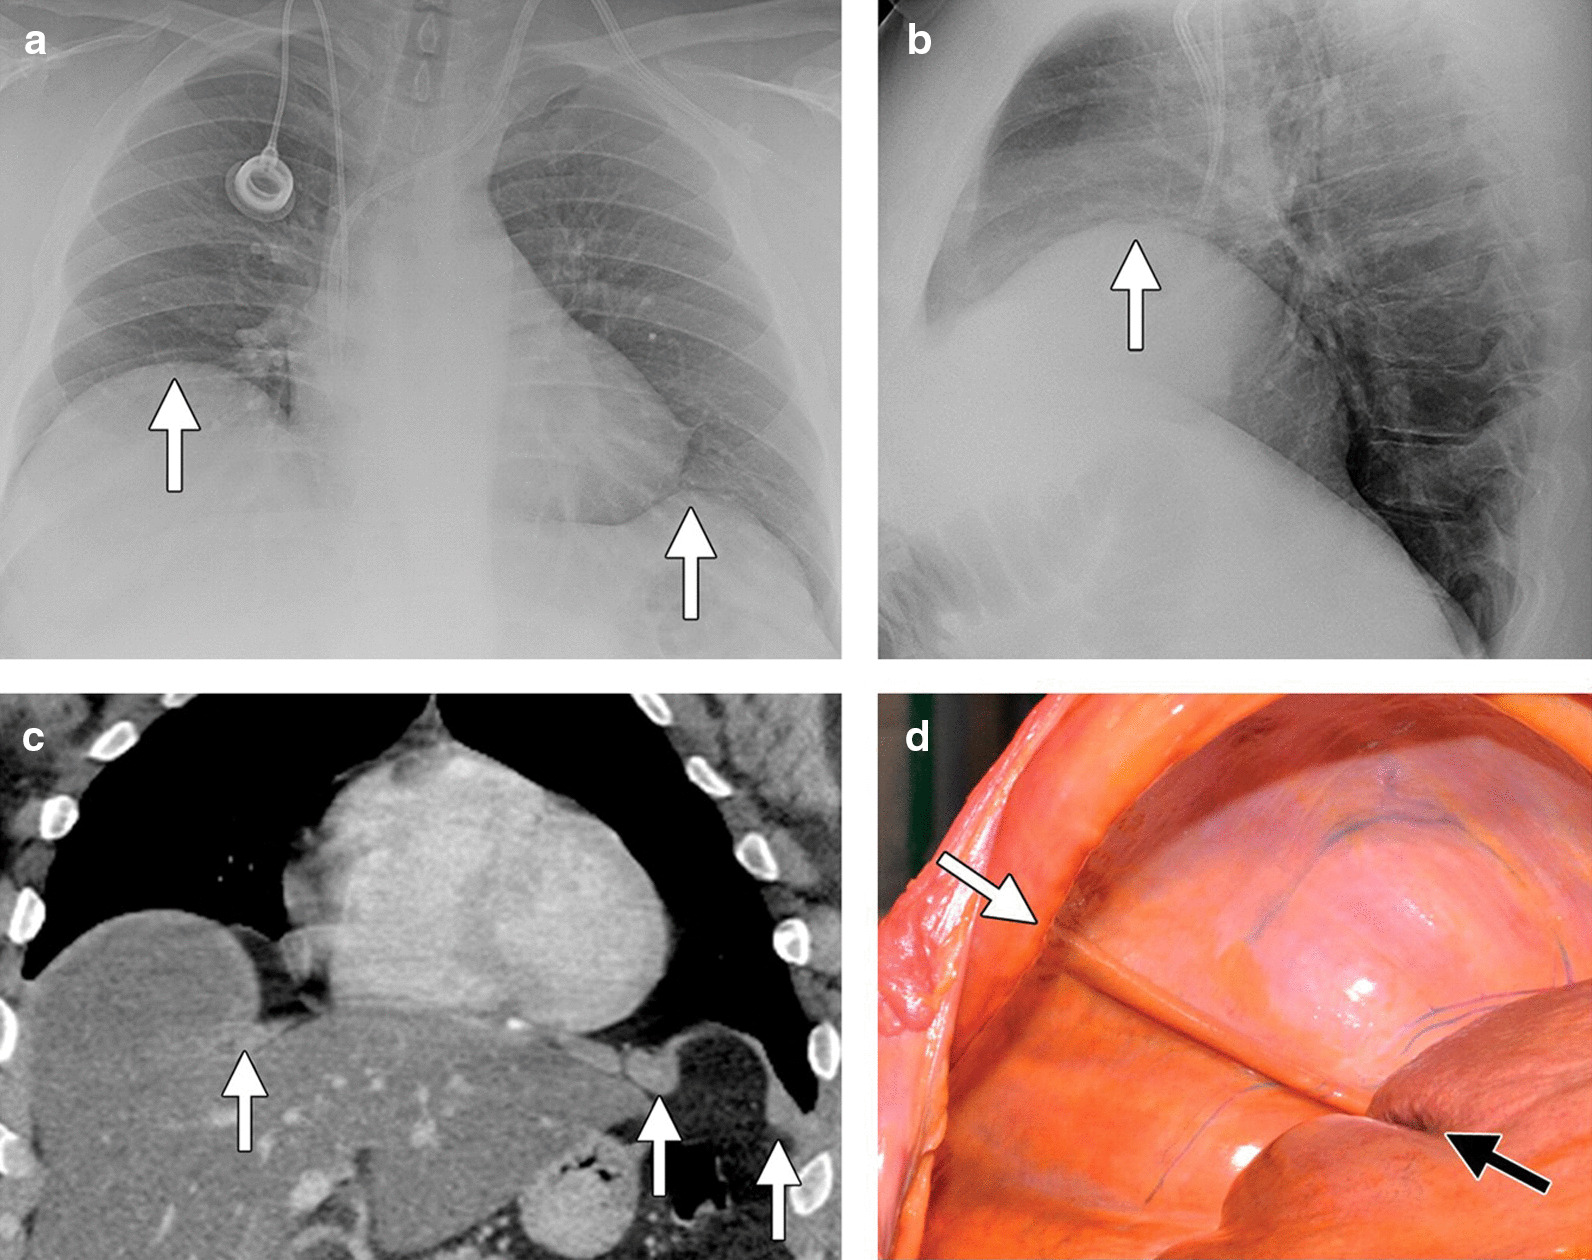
Bilateral hemidiaphragm eventration. Posteroanterior (a) and lateral (b) radiographs demonstrate focal elevation of the right and left hemidiaphragms (white arrows). Axial (c) CT image shows the mushroom appearance of the right and left hemidiaphragms with undercut edges (white arrows). On the right, a portion of the liver occupies the eventration herniation. Eventration (d) on pathology photograph showing the medial aspect (white arrow) of the muscle band and the groove on the liver created by the diaphragm (black arrow). Reproduced with permission from Radiologic Society of North America: Nason et al. Radiographics 2012;32(2):E51–E70
